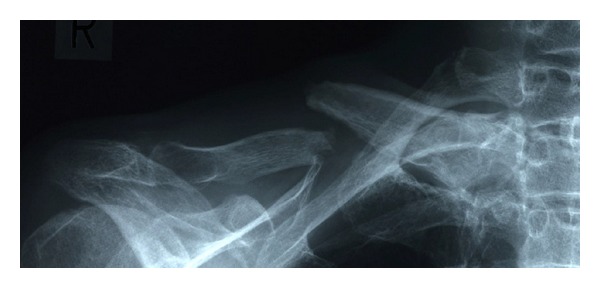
Preoperative radiograph of the fractured right clavicle demonstrating atrophic nonunion.
